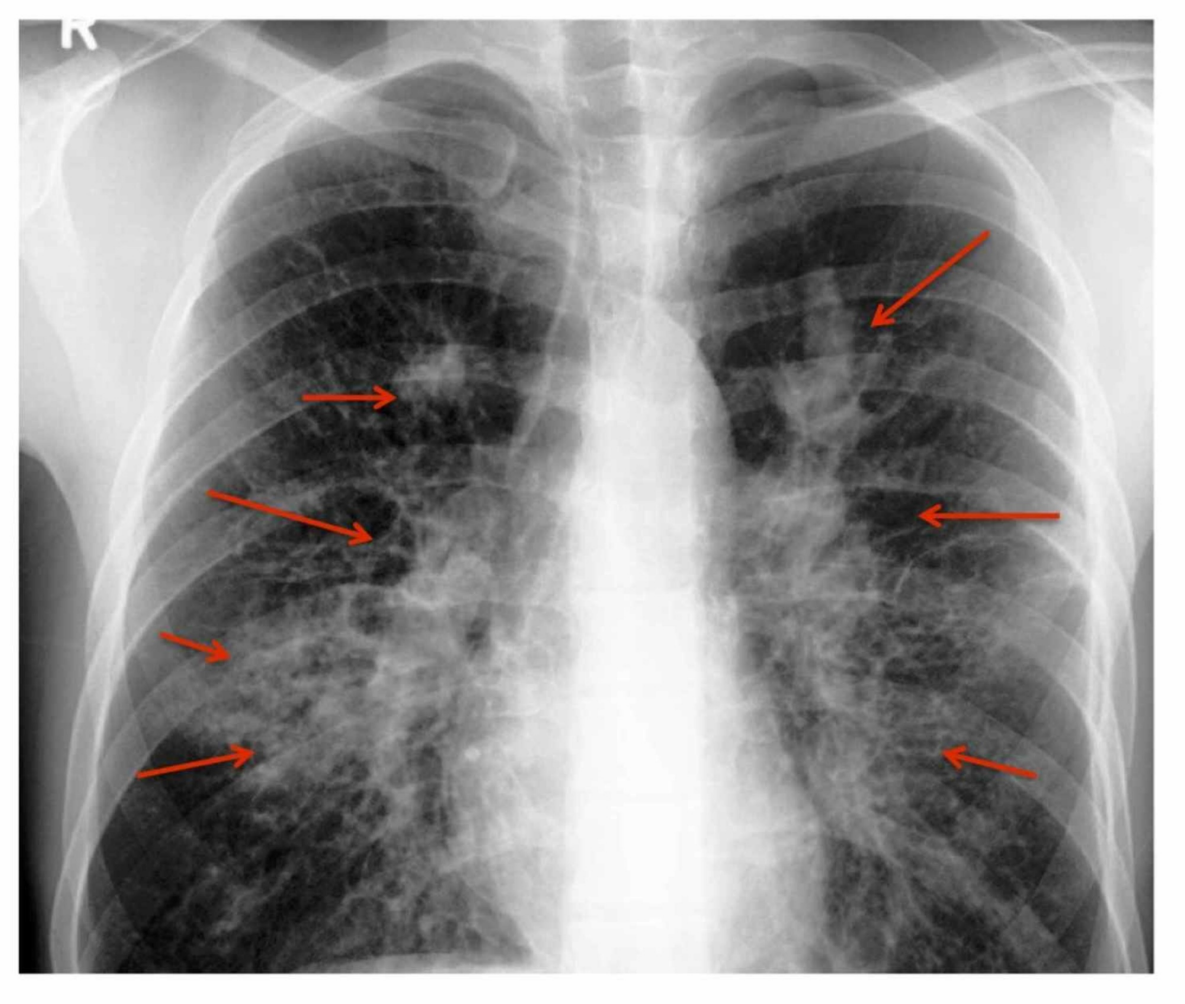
Chest Radiograph of the Same Patient Four Months Later Chest radiograph showed spontaneous resolution of left-sided perihilar opacity with an increase in non-homogenous infiltrates (red arrows) [3].
